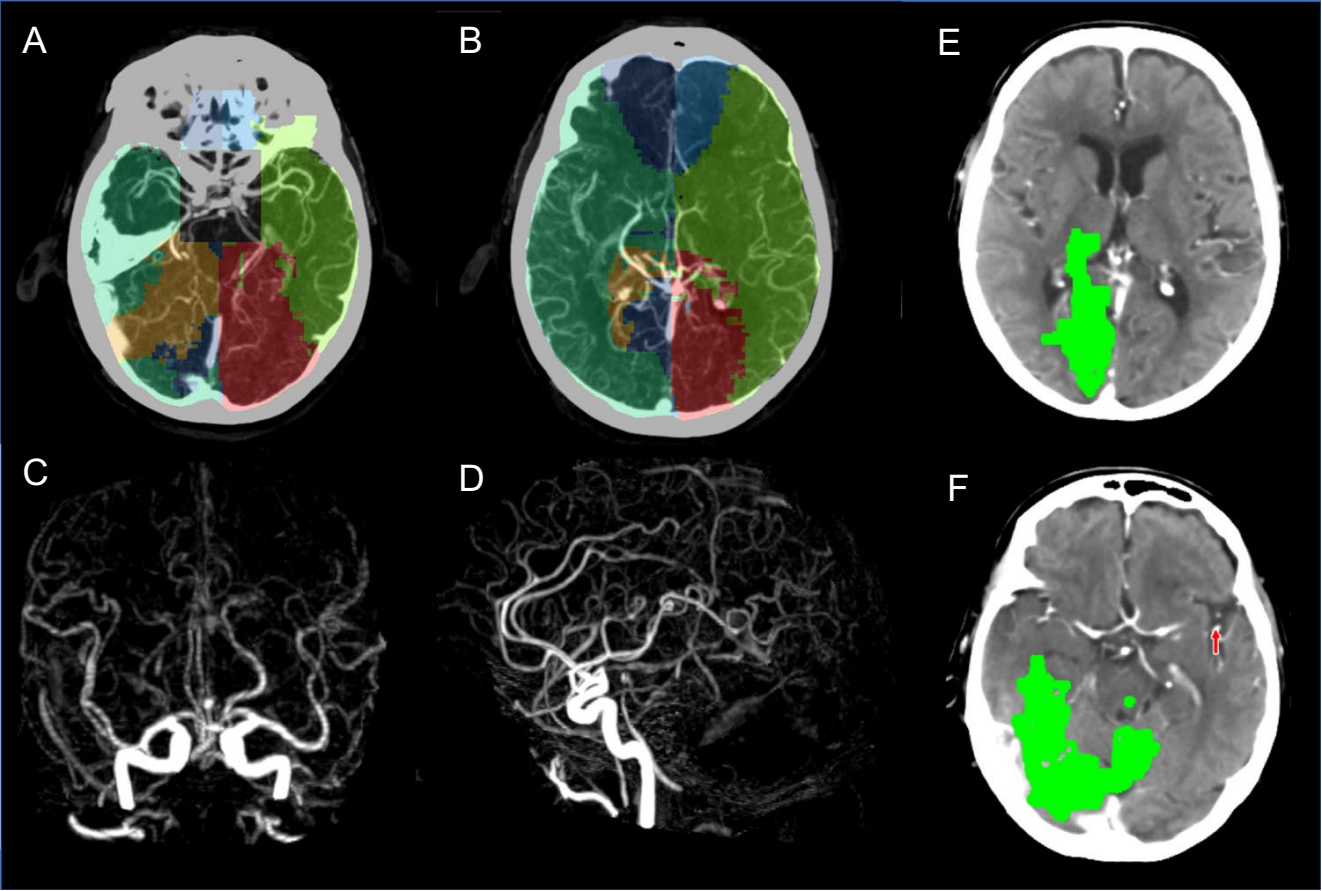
Right PCA occlusion. Occluded pre-communicating segment (P1) of the right PCA. A, B Axial slices of CTA with VTM overlay. C, D MIP CTA demonstrates occlusion of the right PCA. E, F CT Perfusion summary slices. VTM software-derived vascular regions show a reduced right PCA region (yellow) with an increase of both ACA and MCA regions on the ipsilateral side. Notably, the penumbra on CT perfusion within the right PCA territory has a complete absence of CT perfusion core, due to the excellent right MCA collaterals that have been identified on VTM. On CTP there is 141 ml of penumbra (green) and 0 ml of perfusion core (red)
